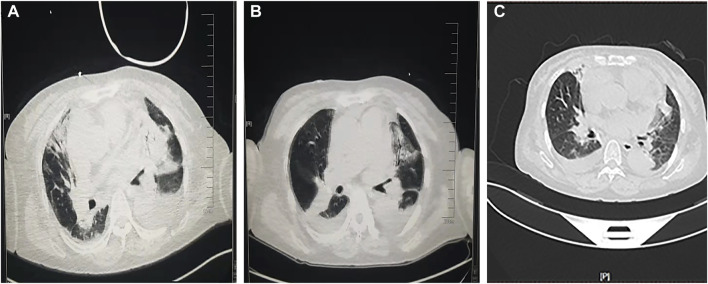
A comparison of pulmonary computed tomography (A) before glucocorticoids administration, (B) after weaning, and (C) before discharge.
